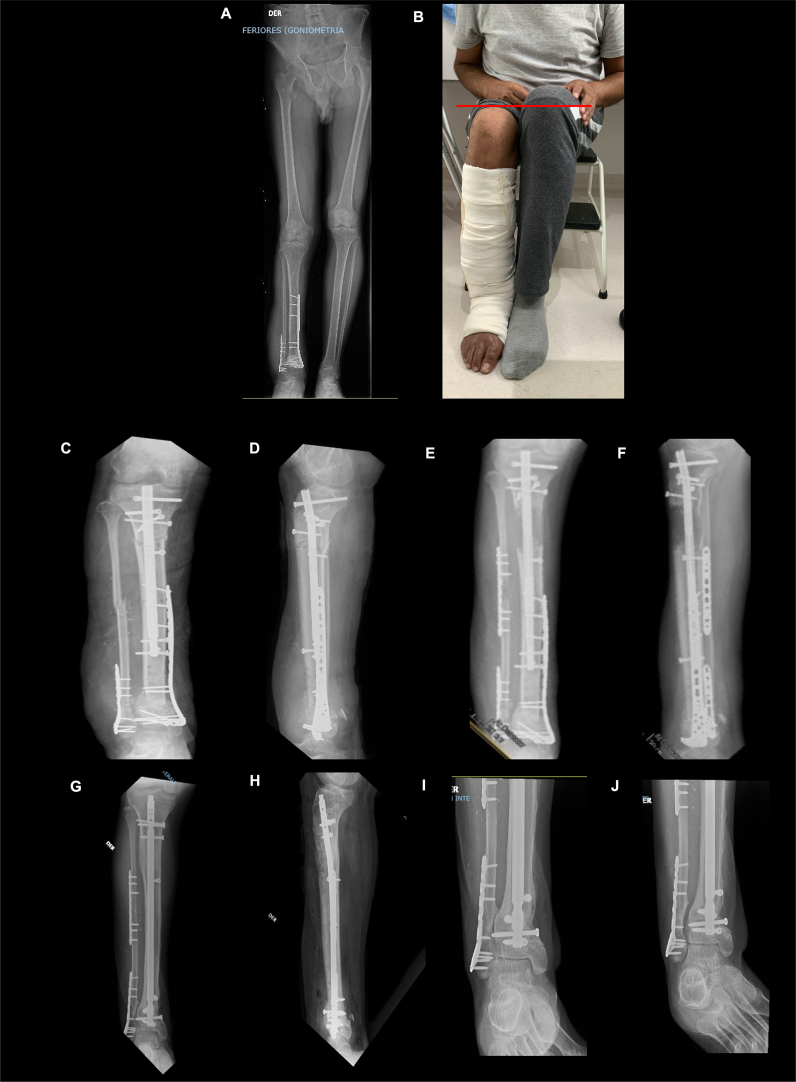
A) Orthoradiography with length discrepancy in lower limbs of 5 cm in relation to the contralateral limb secondary to acute shortening associated with 25 mm of bone defect in the tibial pilon. B) Red line highlights the discrepancy. C–D) X-ray Anteroposterior and lateral PRECICE nail placement with proximal metaphyseal osteotomy. E–F) Stabilization of the fibula with a locking compression plate. G–H): Consolidation of regenerated proximal metaphyseal. I–J): Consolidation of Masquelet distal tibia.
